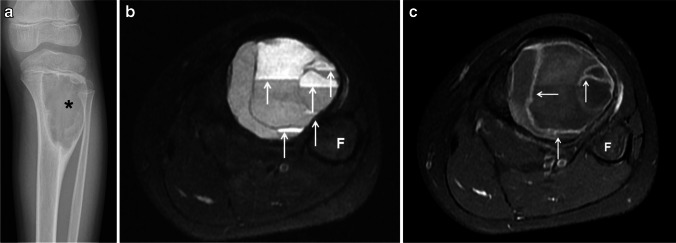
Type III aneurysmal bone cyst of the tibia in a 10-year-old girl. a An anteroposterior radiograph of the tibia shows an eccentric, well-defined, juxtaphyseal, lytic and expansile lesion (*asterisk*) involving the proximal tibial metadiaphysis. b An axial fat-suppressed T2-weighted magnetic resonance (MR) image of the leg shows fluid-fluid levels throughout the lesion (*arrows*). c A fat-suppressed, contrast-enhanced, T1-weighted MR image of the lesions shows thin, enhancing septations (*arrows*). *F* fibula
